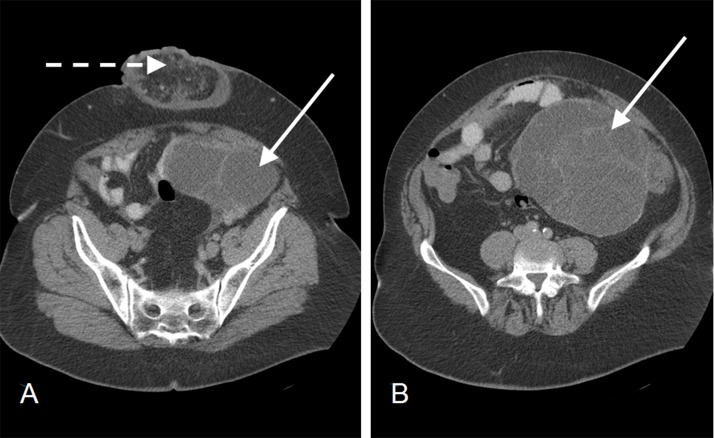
Computed tomography of the pelvis demonstrating a multiloculated mass (solid arrow) arising from the left adnexa and an incisional hernia containing omentum (dashed arrow)
